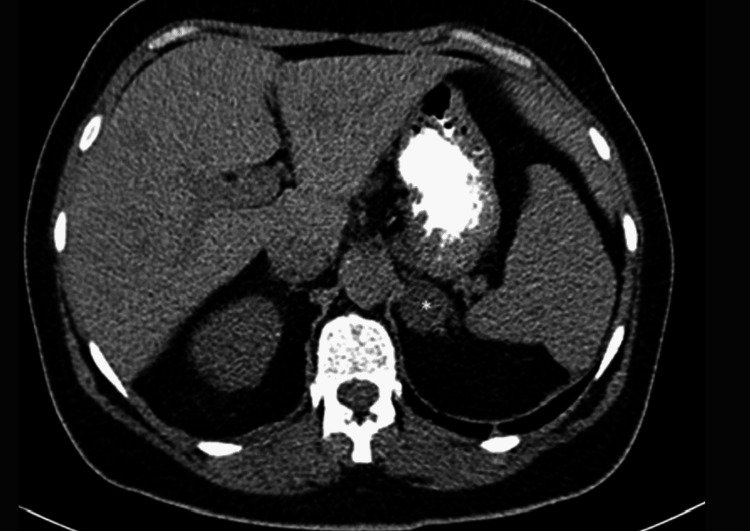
Abdominal CT showing an adenoma of the left adrenal gland (* marking the adenoma) - axial plan.
